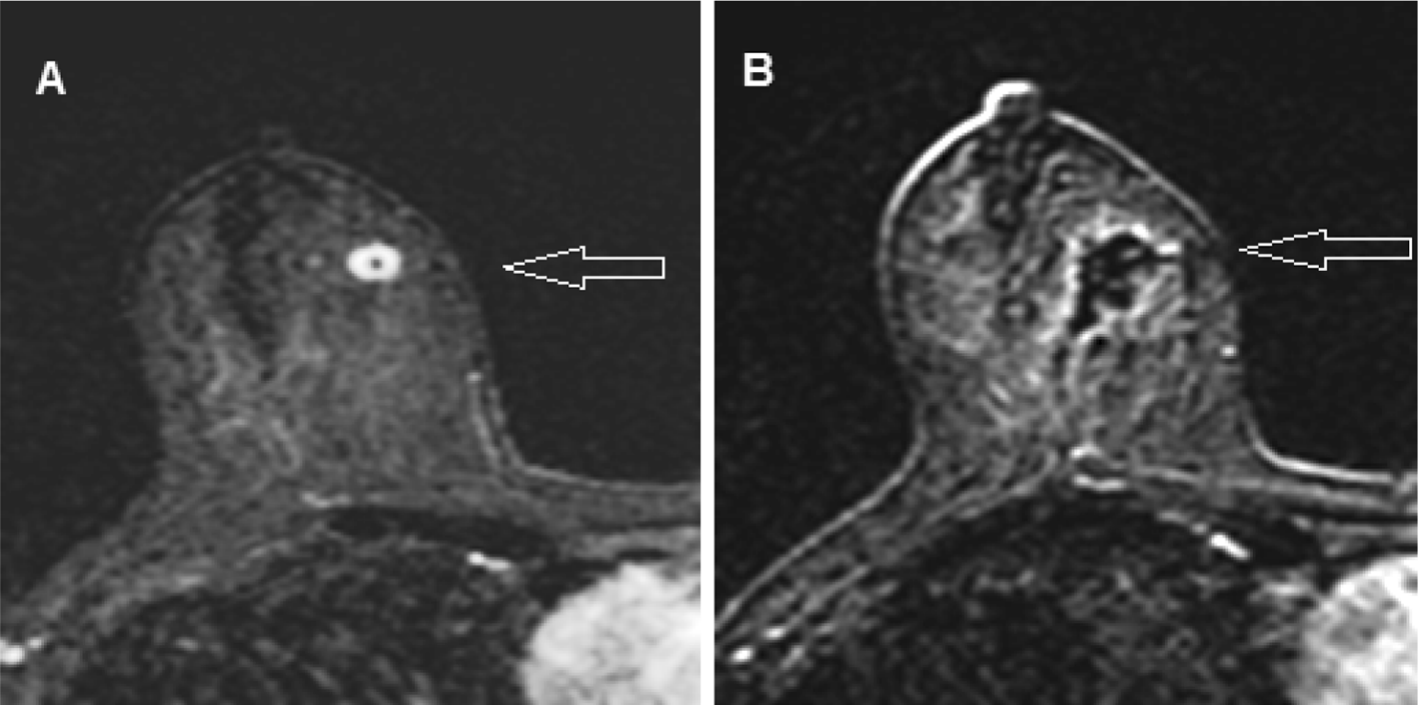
Example of magnetic resonance imaging (MRI). a Coronal gadolinium-enhanced, fat-suppressed, pre-ablation MRI image of an 11-mm grade 1 invasive ductal breast carcinoma (IDC). b Coronal MRI obtained 28 days after PLA showing ablation zone with no enhancement at the site of the cancer
